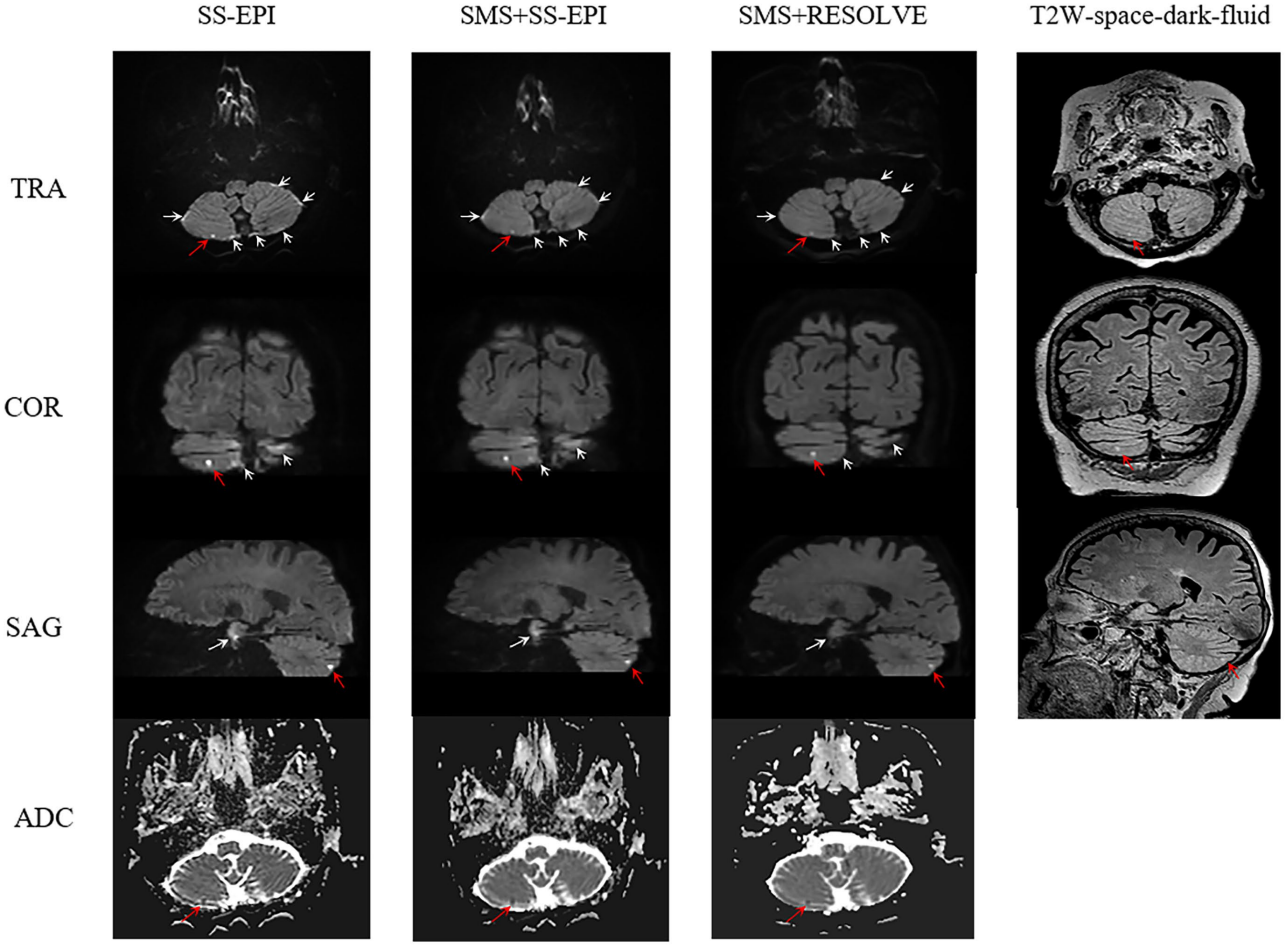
Female, 75 years old. On the SS-EPI-ISO DWI sequence (red arrow), punctate high signals in the right cerebellar hemisphere are indistinguishable from artifacts (white arrow). The apparent diffusion coefficient (ADC) shows low signals, with visibility score of 4 point, and artifacts and geometric distortions score of 2 point (white arrow), overall image quality score of 4 point. On the SMS + SS-EPI-ISO DWI sequence, faint punctate high signals are vaguely visible in the right cerebellar hemisphere, ADC shows low signals, with visibility score of 3 point, and artifacts and geometric distortions score of 3 point, Overall image quality score of 4 point. On the SMS + RESOLVE-ISO DWI sequence, a faint high signal is visible in the right cerebellar hemisphere. ADC shows low signal, with visibility score of 2 point, and artifacts and geometric distortions score of 2 point, overall image quality score of 5 point. The T2W-space-dark-fluid sequence reveals punctate high signals corresponding to the right cerebellar hemisphere.
